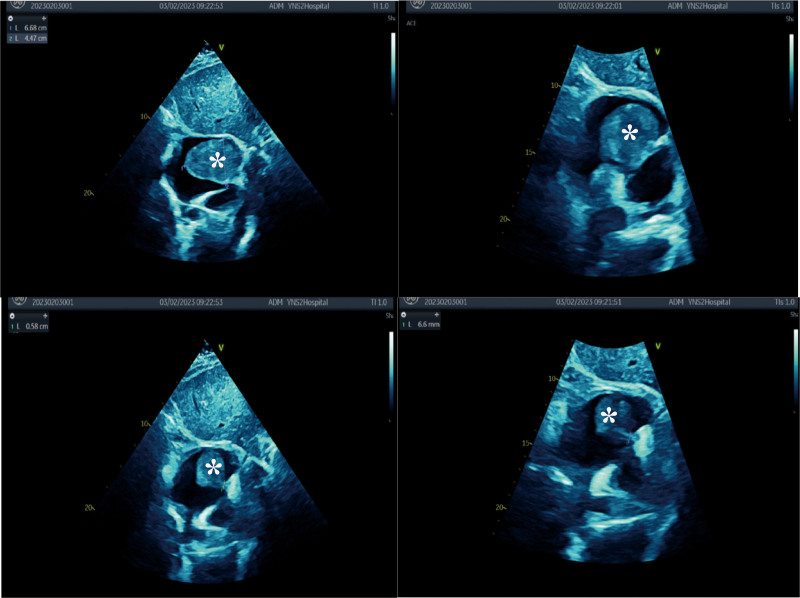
Three-dimensional transthoracic echocardiography showed a 66.8 mm × 44.7 mm voluminous mass (asterisks) in the right atrium.
